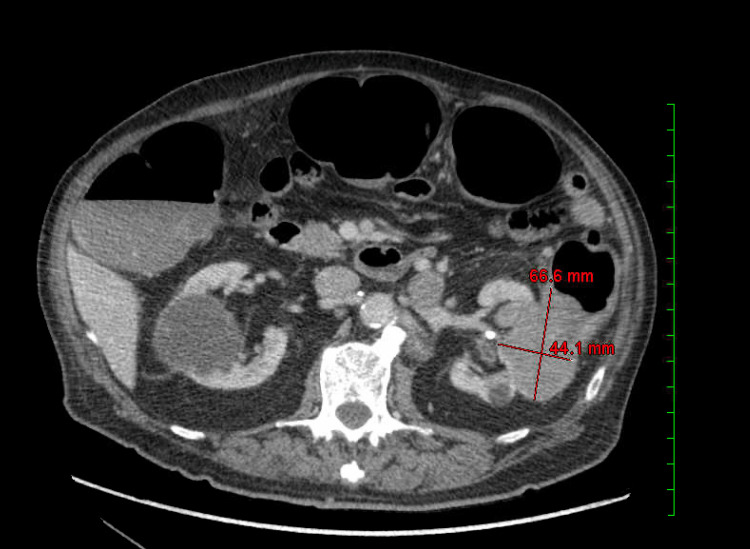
Abdominal CT scan The abdominal CT scan found that the patient's known left renal mass is showing further enlargement, now measuring up to 6.6 cm and extending into the adjacent descending colon.
